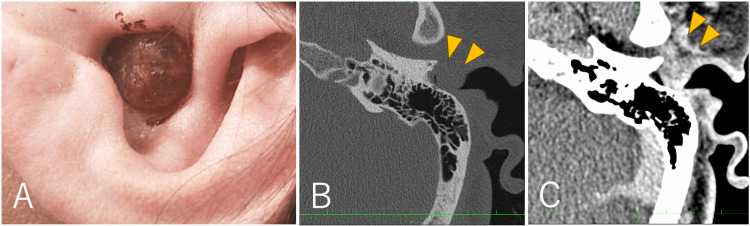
Preoperative photograph and CT images (A) Preoperative photograph of the left auricle showing a brownish mass occupying the entrance of the external auditory canal. (B) Temporal bone CT revealed a 17-mm mass in the cartilaginous portion of the external auditory canal (arrowhead) without obvious invasion into the bony portion. (C) Contrast-enhanced CT demonstrated uniform enhancement of the mass with partial enhancement of the temporomandibular joint capsule (arrowhead).
